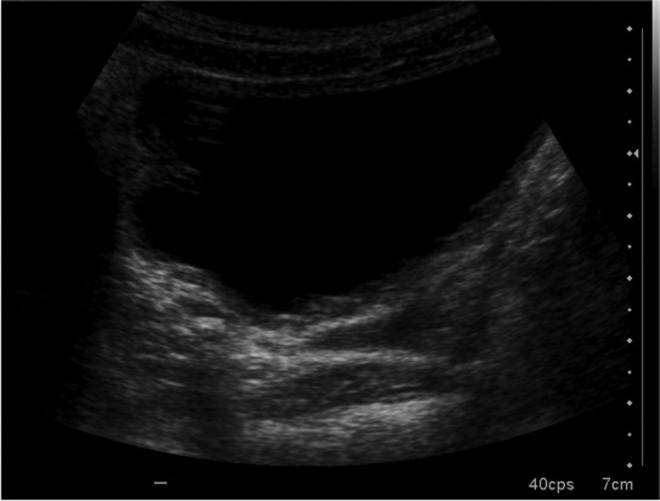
Ultrasonography in which a hyperechoic area is detected on the right distal ureter.
